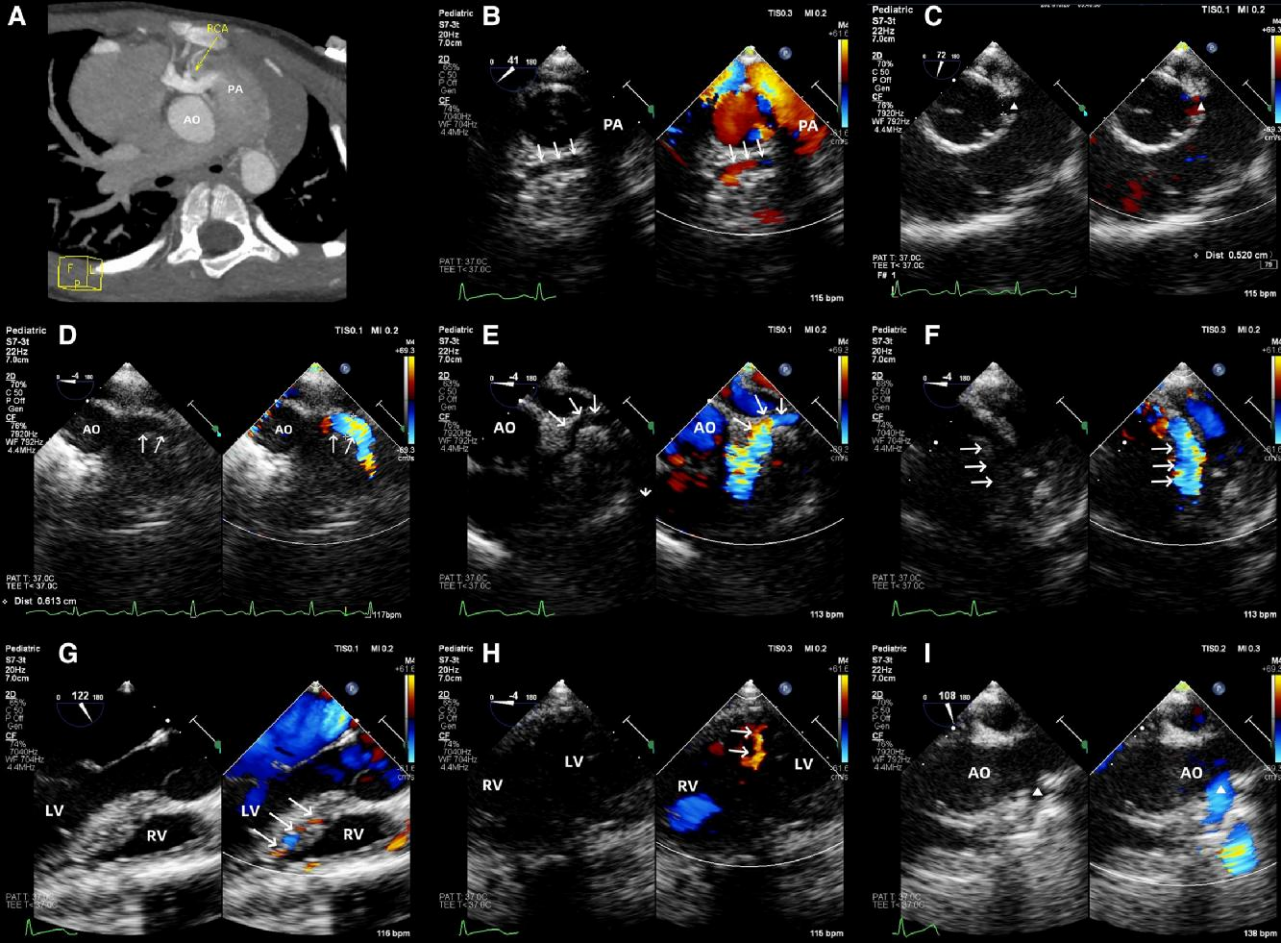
Transoesophageal echocardiography was utilized to assess an anomalous origin of the RCA from the MPA. (*A*) CCTA reveals the RCA arising from the MPA (arrow). (*B*) In the mid-oesophageal aortic valve short axis view, the RCA is observed emerging from the MPA (arrows). (*C*) Mid-oesophageal aortic valve short axis view shows the dilated LCA arising centrally from the left sinus of Valsalva (triangle). Based on mid-oesophageal five-chamber view, the dilated LMCA (*D*) was visualized by slight adjustment of the probe. Following the course of the LMCA, the dilated LCX (*E*) and LAD (*F*) are identified (arrows). (*G*) Mid-oesophageal long axis view shows flow signals within the ventricular septum (arrows). (*H*) Transgastric mid-short-axis view demonstrates flow signals within the ventricular septum (arrows). (*I*) Post-operative TEE examination reveals the re-implanted RCA in the mid-oesophageal aortic valve long axis view (triangle). AO, aorta; RCA, right coronary artery; PA, pulmonary artery; LV, left ventricle; RV, right ventricle; CCTA, coronary computer tomography angiography; LCA, left coronary artery; LMCA, left main coronary artery; LCX, left circumflex coronary artery; LAD, left anterior descending artery.
